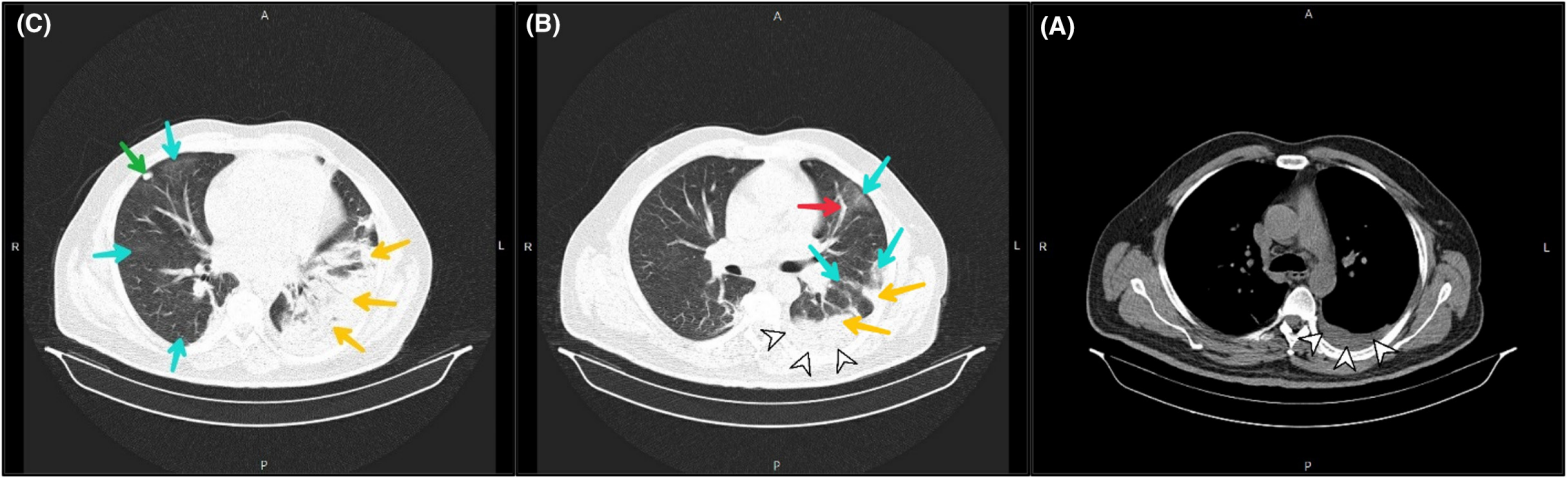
Mediastinal (A) and parenchymal (B, C) view of spiral CT scan of the patient's chest 2 weeks after admission. (A) in mediastinal view mild pleural effusion in the left lung (white arrowheads) is evident; (B) Several focal ground‐glass opacities with more intensity in the left lung (blue arrows), atelectatic band in the left lung (red arrow), alveolar consolidation areas of left lower lobe (yellow arrows), mild pleural effusion in the left hemithorax is seen in parenchymal view (white arrows); (C) Large areas of left lower lobe consolidation (yellow arrows), several focal peripheral ground‐glass opacities in the right lung (blue arrows), a nodule in the lateral segment of the middle lobe of the right lung (green arrow) is seen
